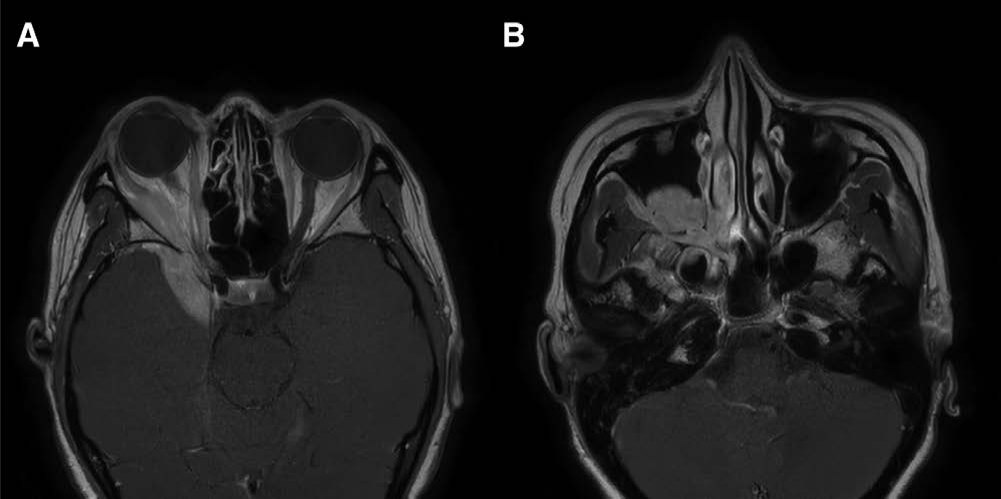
Axial scans of T1-weighted multipoint Dixon sequence in magnetic resonance imaging of the tumor. The tumor shows high signal intensity in, (A) the right retrobulbar area and the middle cranial fossa and (B) the right maxillary sinus.
